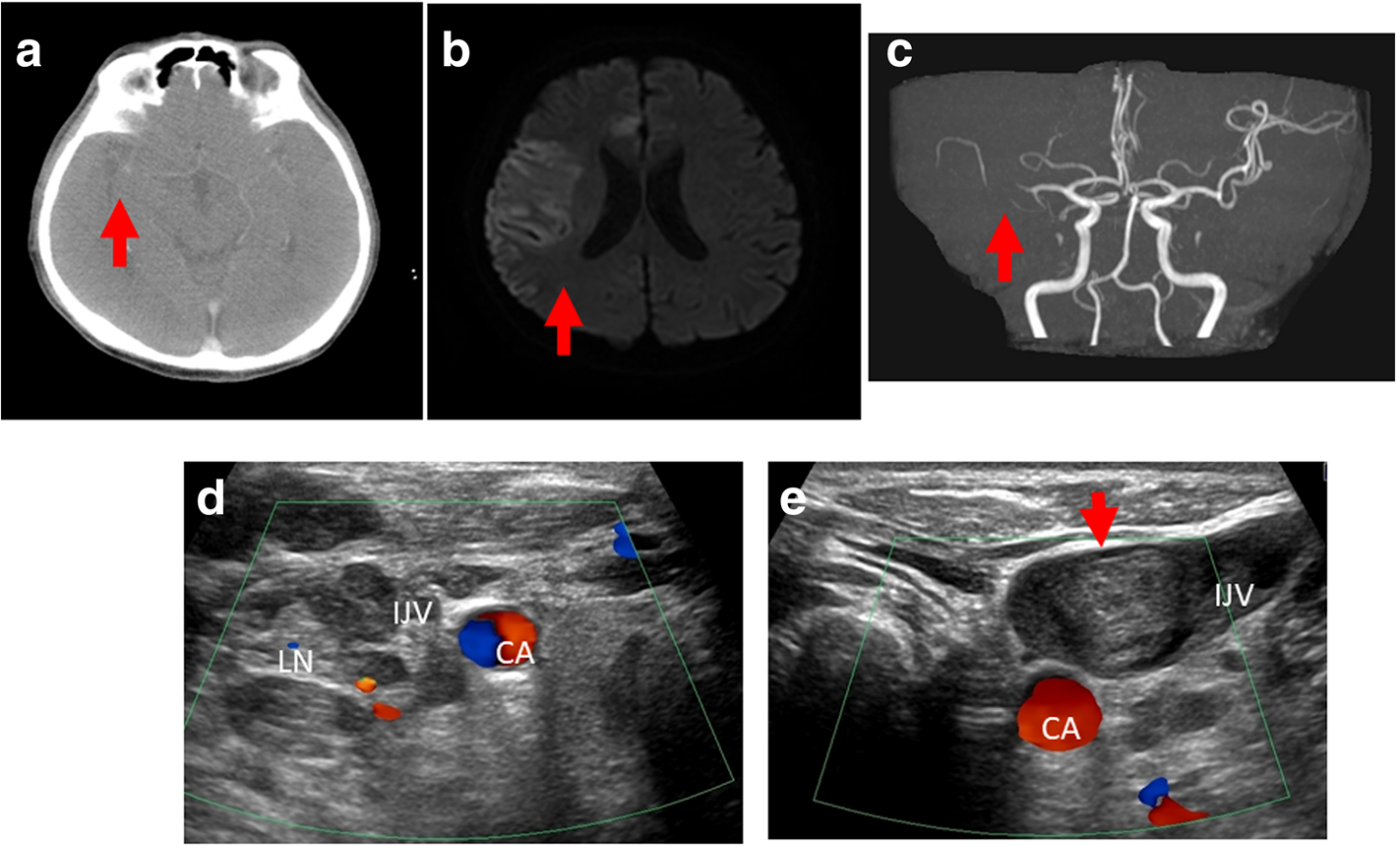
Patient computed tomography scan images after onset of aphasia and loss of consciousness. a Scattered hyperdense curvilinear areas (*red arrow*) suggestive of developing petechial hemorrhage in the region of the right middle cerebral artery. b Diffusion-weighted image showed a scattered lesion (*red arrow*) affecting the cortical part supplied by the right middle cerebra artery with corresponding deficit. c Head magnetic resonance angiography showed attenuated flow-related signal in middle cerebral artery beyond the M1 segment, while its superior division was not visible (*red arrow*). d A Doppler ultrasound scan of the neck revealed that the right internal jugular vein was compressed by metastatic lymph nodes. e A thrombosis was detected in the left internal jugular vein (*red arrow*). *CA* carotid artery, *IJV* internal jugular vein, *LN* metastatic lymph node
